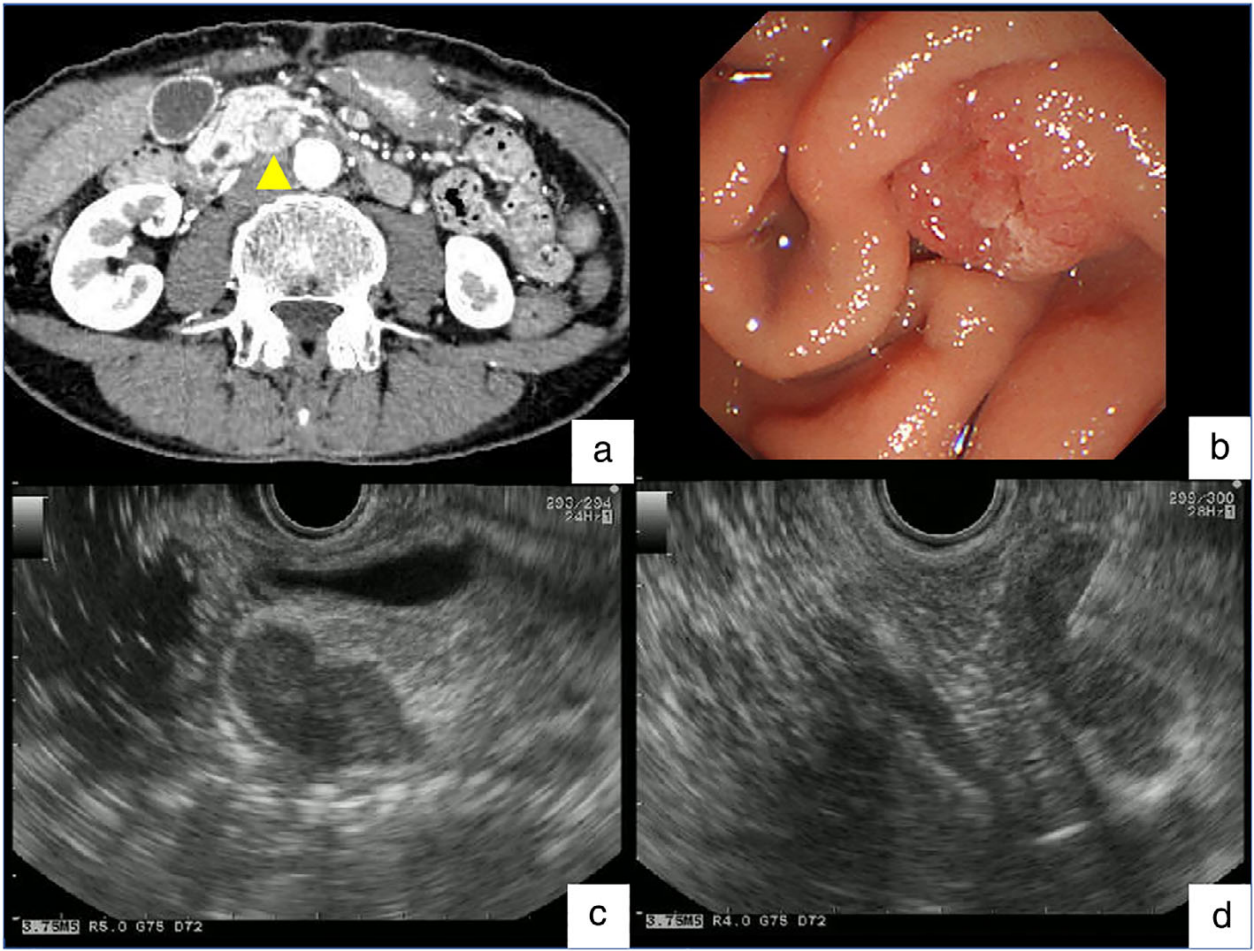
(a) Contrast‐enhanced computed tomography (CT) showing an 18‐mm enlarged lymph node (LN), adjacent to the pancreatic head (arrowhead). (b) Side‐viewing duodenoscopy, revealing no apparent abnormality on the papilla of Vater. (c) Observation with endoscopic ultrasound (EUS) using water immersion technique, revealing a hypoechoic mass with irregular contour (enlarged LN), which ruled out ampullary cancer of the nonexposed protruded‐type. (d) EUS‐guided fine needle aspiration with 22‐gauge needle for the enlarged LN.
